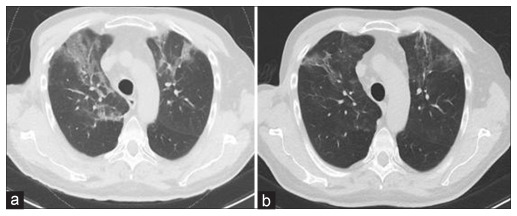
Imaging of PJP infection. Computed tomography scan of the thorax showing diffuse interstitial infiltrates bilaterally correlating with (a) Pneumocystis jiroveci pneumonia and (b) clearing of these infiltrates after 2 weeks of trimethoprim/sulfamethoxazole. PJP: Pneumocystis jiroveci pneumonia.
